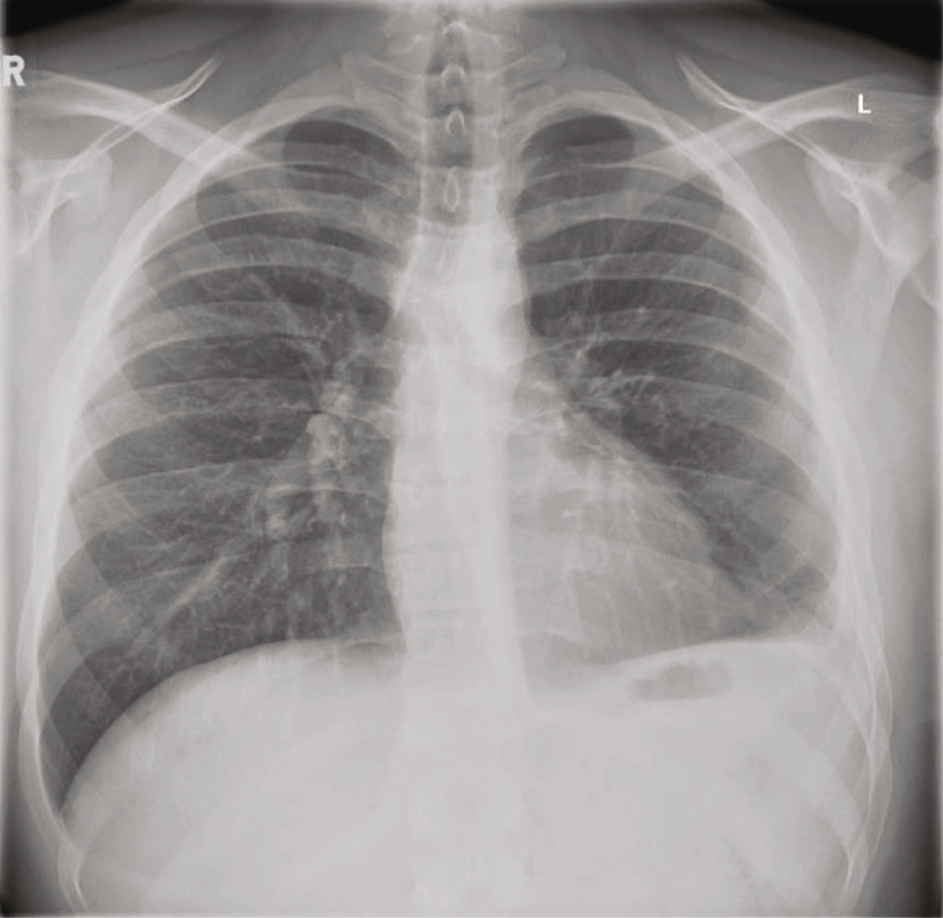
Chest X-ray taken at 2 weeks postdischarge showing clear lung fields and minimal blunting of the left costophrenic angle.
